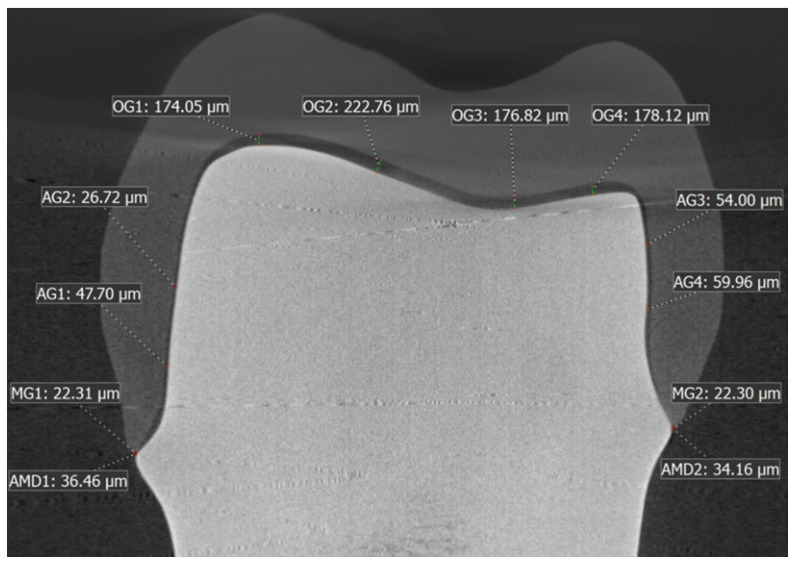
Representative image of the crown-master metal die bucco-lingual section of the nano-CT 3D scan model showing the internal and marginal gap width measurements.
